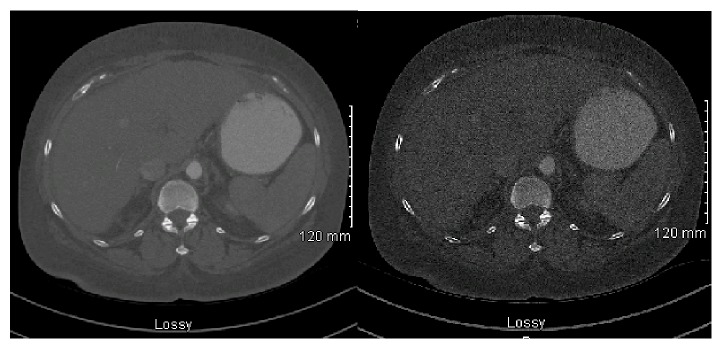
Abdominal Computed Tomography (CT), 2015.
